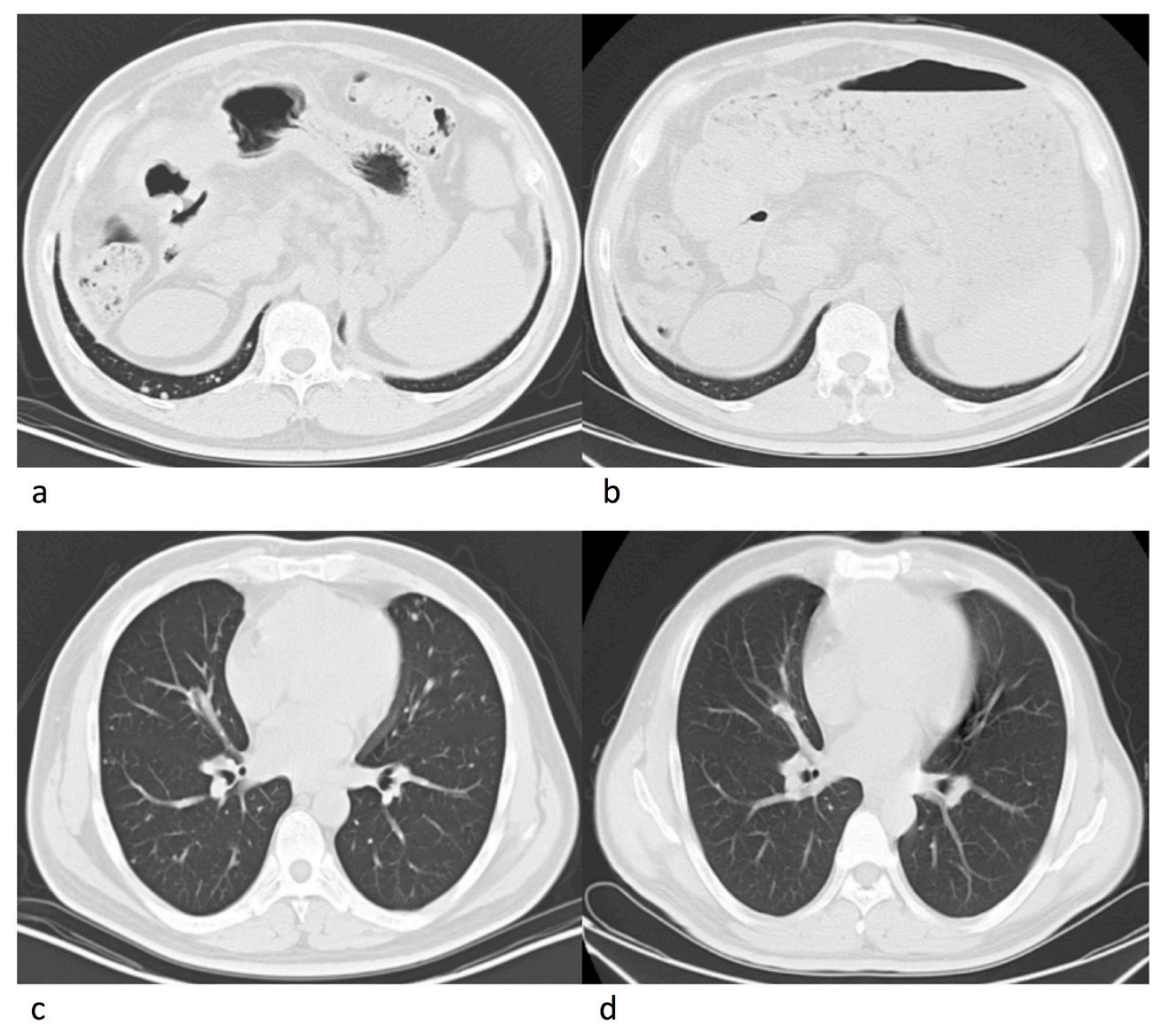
Radiographies of a 43-year old male patient Tumors in lungs disappeared after 8 cycles of nivolumab. a., c. Pre-treatment. b., d. Post-treatment.
